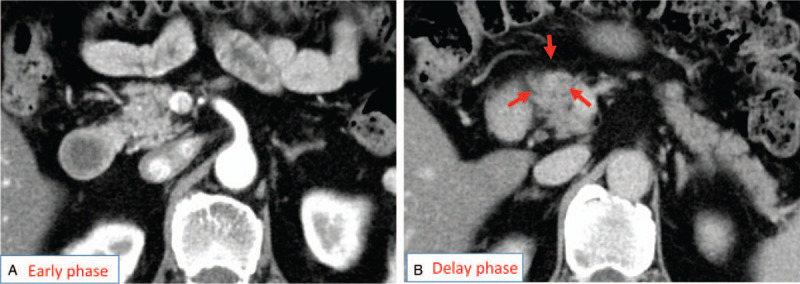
Abdominal computed tomography (dynamic study, sliced in 5 mm) revealed no abnormal mass in the early phase (A), but there was a weak enhanced round mass (arrows) detected in the pancreas head at the delay phase (B).
